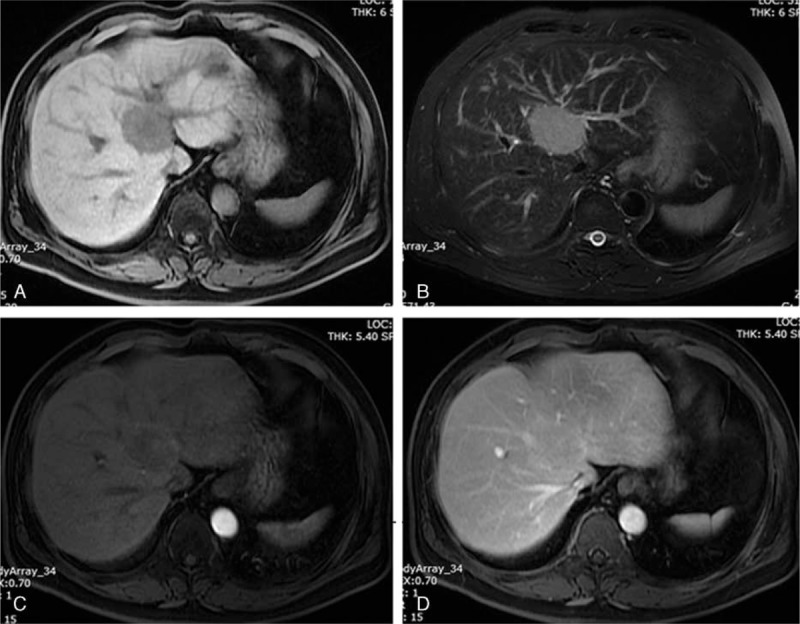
Enhanced magnetic resonance imaging findings. The mass was demonstrated low signal intensity on T1-weighted imaging (A) and slightly high signal intensity on T2-weighted imaging (B). The mass was slightly and peripherally enhanced in the arterial phase (C) and portal phase (D).
